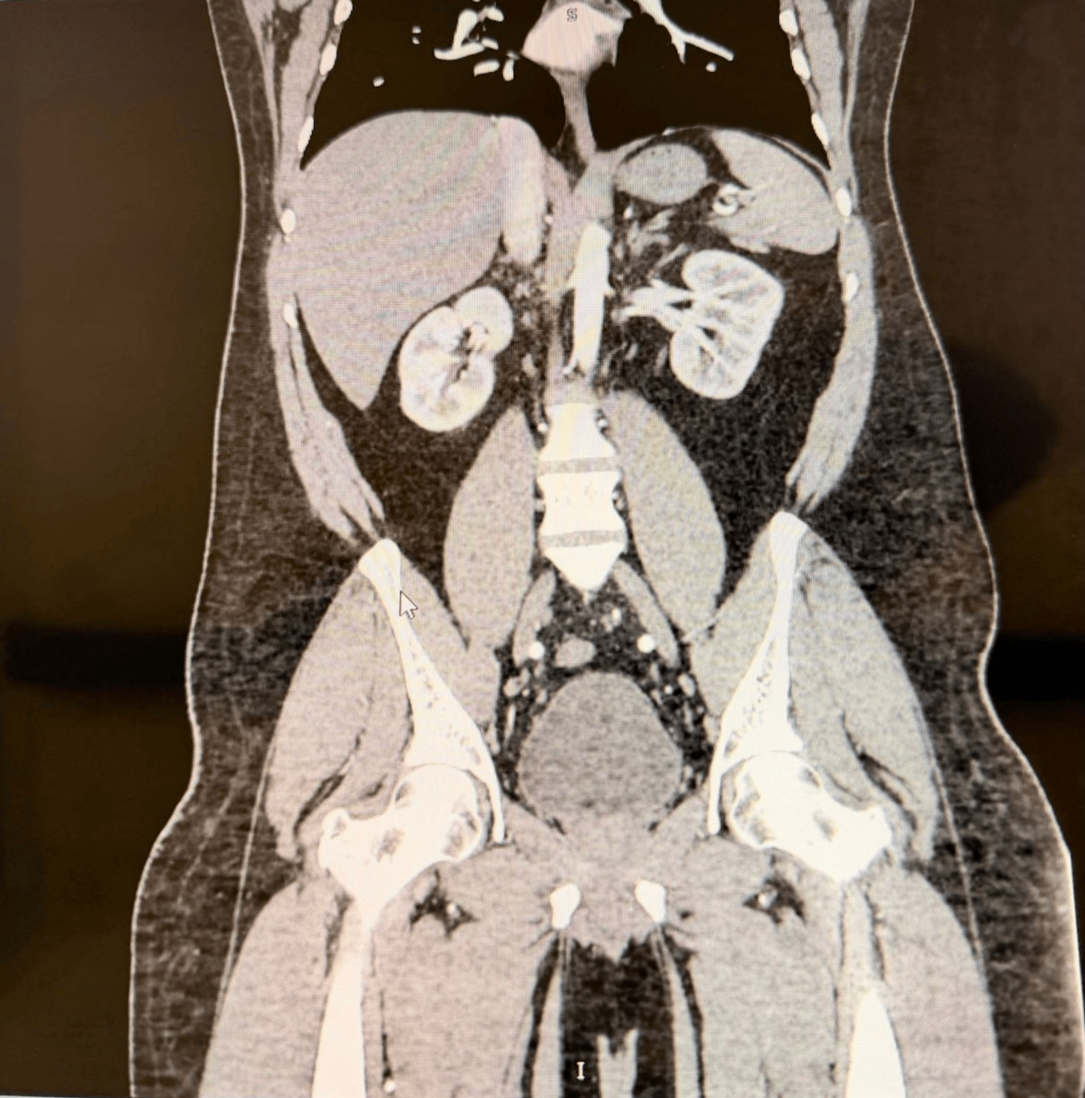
Normal CT Angiography of Abdomen and Pelvis Contrast-enhanced CT angiography of the abdomen and pelvis demonstrating normal abdominal vasculature with preserved vessel caliber and enhancement, without evidence of aneurysmal dilatation, stenosis,dissection or mural irregularity.
